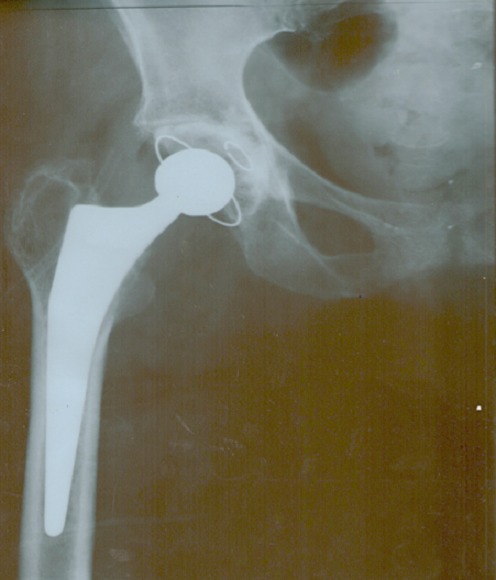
Radiographie montrant une pseudarthrose après traitement par vissage chez un homme de 54 ans
